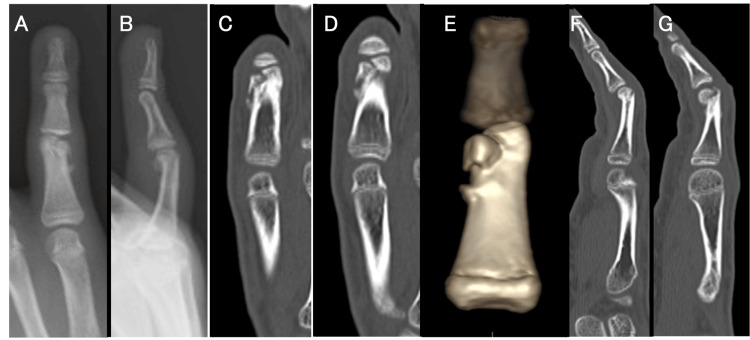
Preoperative radiographs and CT images. Radiographs (A, B) and CT scan images (C, D, E, F, G) showed that the ulnar condyle was proximally shortened, the articular step-off was 2 mm, and the radial head demonstrated 10° of ulnar deviation.
